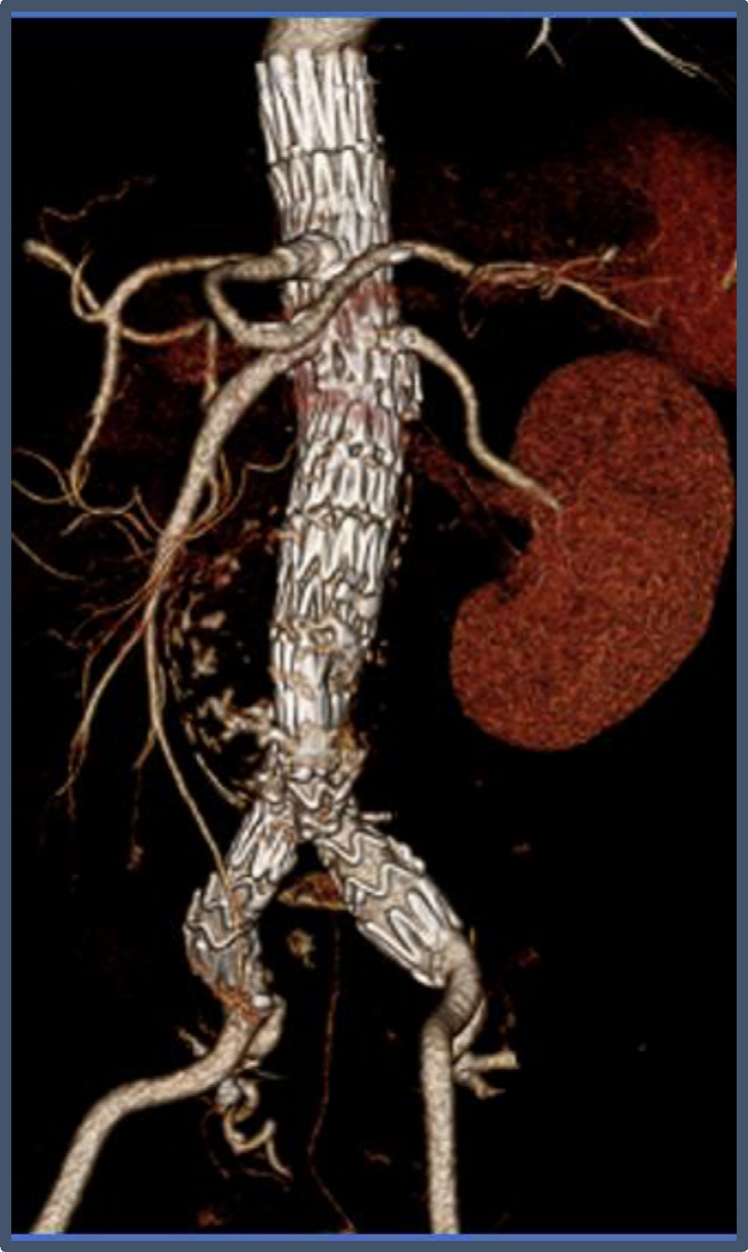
Three-dimensional reconstruction of a postoperative computed tomography scan performed in follow-up of the final graft in situ.
